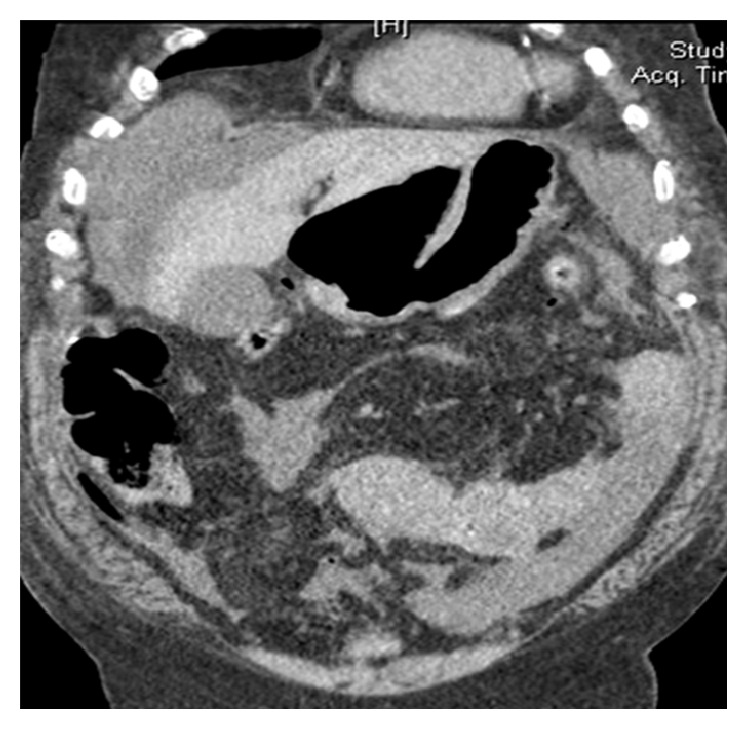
Computed tomography scan of abdomen showing discontinuity of the anterior wall of the gastric antrum with pneumoperitoneum.
